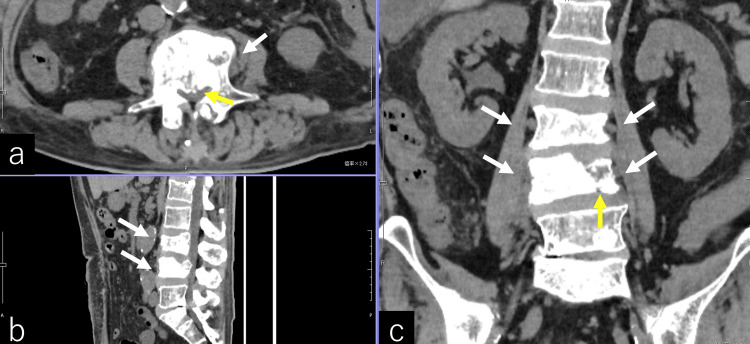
Abdominal plain CT Findings included osteosclerosis of the L4 vertebra (a, white arrow) and spinal canal compression (a, yellow arrow). Sagittal view demonstrated osteosclerosis of L3–L4 (b, white arrows). Coronal view revealed osteosclerosis of the L3–L4 vertebrae with bilateral extension toward the psoas muscles (c, white arrows). A partial compression fracture of the L4 vertebra was also noted (c, yellow arrow).
